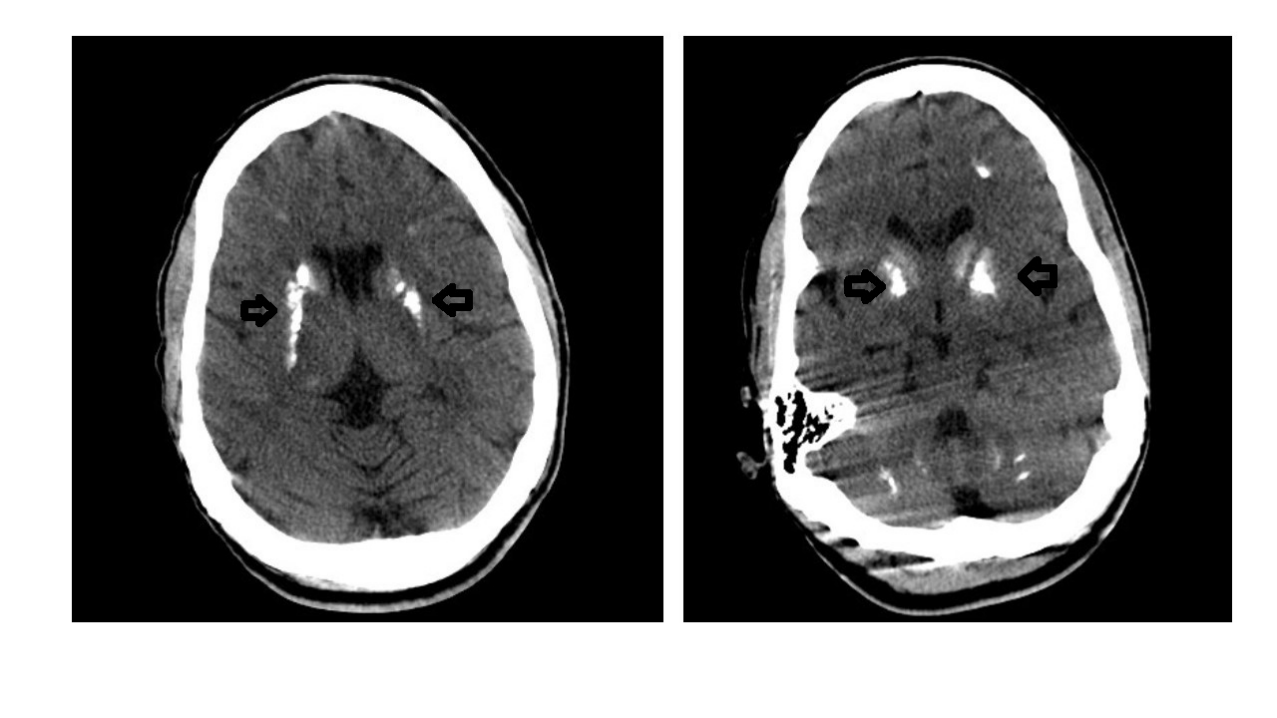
CT head Bilateral basal ganglia calcification as shown by the arrows.
